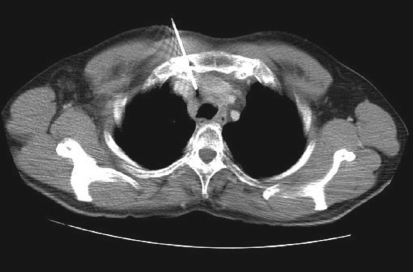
Trans-sternal approach for biopsy of pre-tracheal node in a treated case of renal cell carcinoma on follow up. PET-CT showed hypermetabolism in this node. Biopsy results revealed tuberculous lymphadenitis
